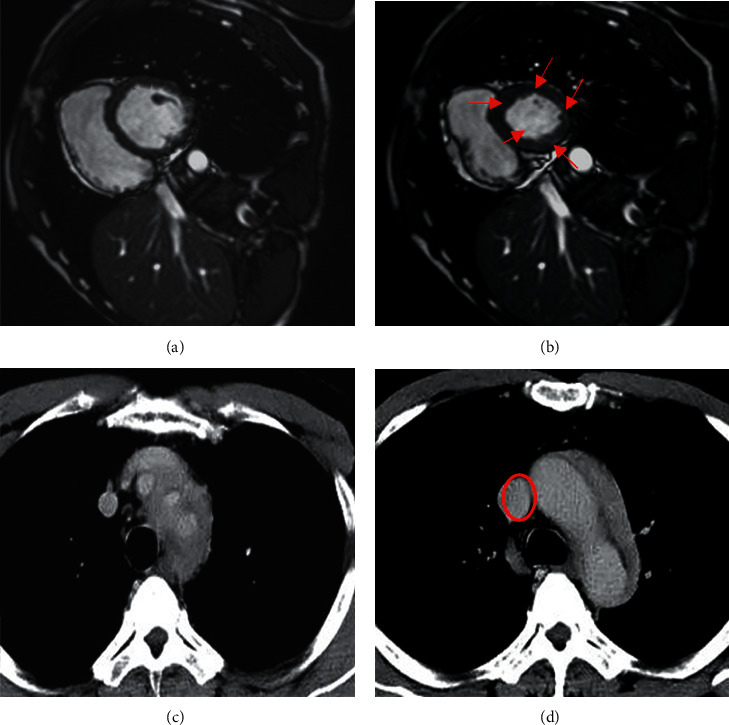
Heart MRI image manifestations. (a) Male, 28 years old, MRI image of normal right ventricle; (b) male, 30 years old, MRI image of right ventricle of PH patients; (c) female, 46 years old, MRI image of normal pulmonary aorta; (d) female, 50 years old, MRI image of pulmonary aorta of PH patients.).
